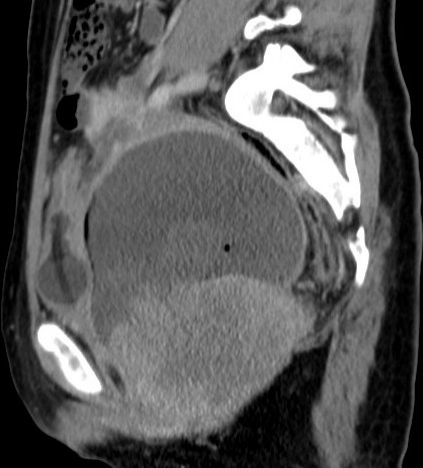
Oblique sagittal view of the pelvis via computed tomography scan showing a large heterogeneous mass in the upper vagina causing obstruction of the os and leading to hydrometra. The urinary bladder is displaced anteriorly and a self-retaining catheter is seen within it.
